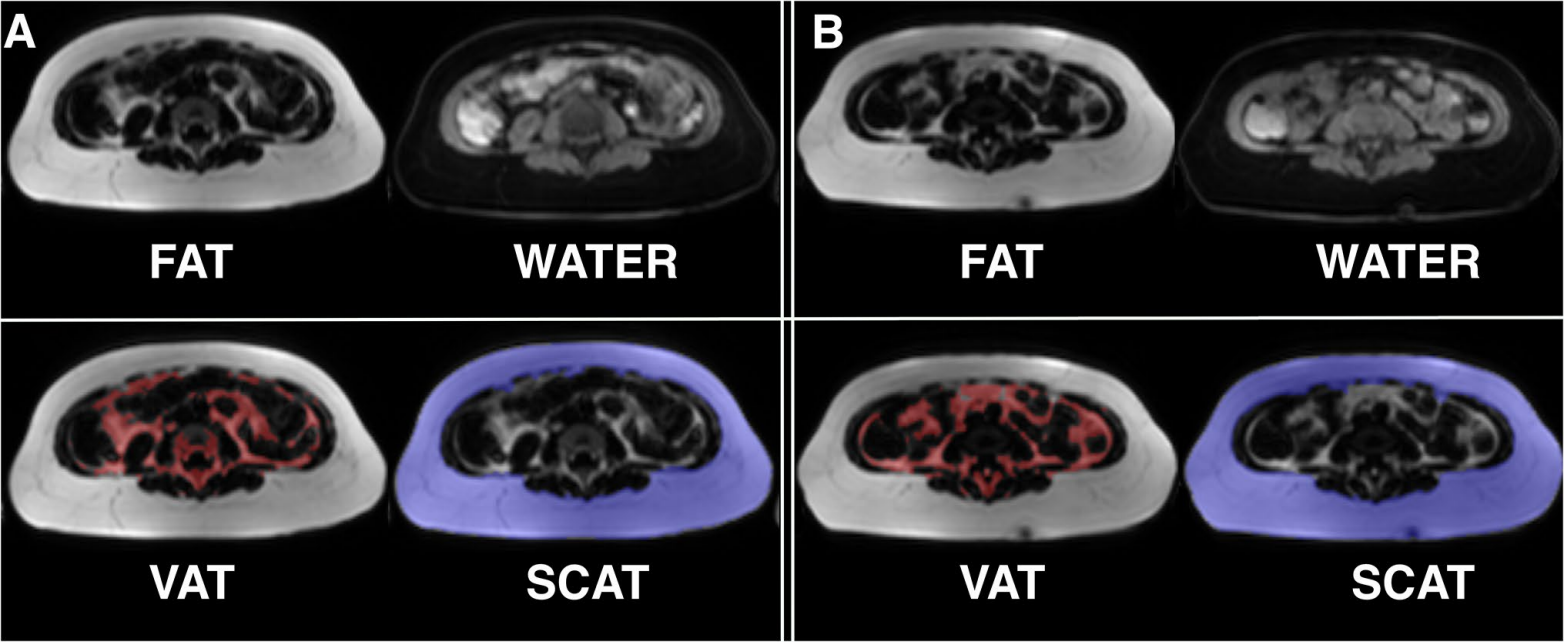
MRI-based segmentation in human melanoma patients treated with an immune checkpoint inhibitor. Representative example for Dixon-based whole-body segmentation of a 64-year-old melanoma patient with PD-1-mAbs therapy at baseline (a) and with progressive disease after 3 months of CIT (b), axial slices of the lumbar region are given. Fat and water MRI images (top row) as well as delineated segments of visceral adipose tissue (VAT, bottom left with VAT indicated in red) and subcutaneous adipose tissue (SCAT, bottom right with SCAT indicated in blue) are shown. Total fat was reduced by − 26% after 12 weeks, body weight was reduced by − 7.2%
